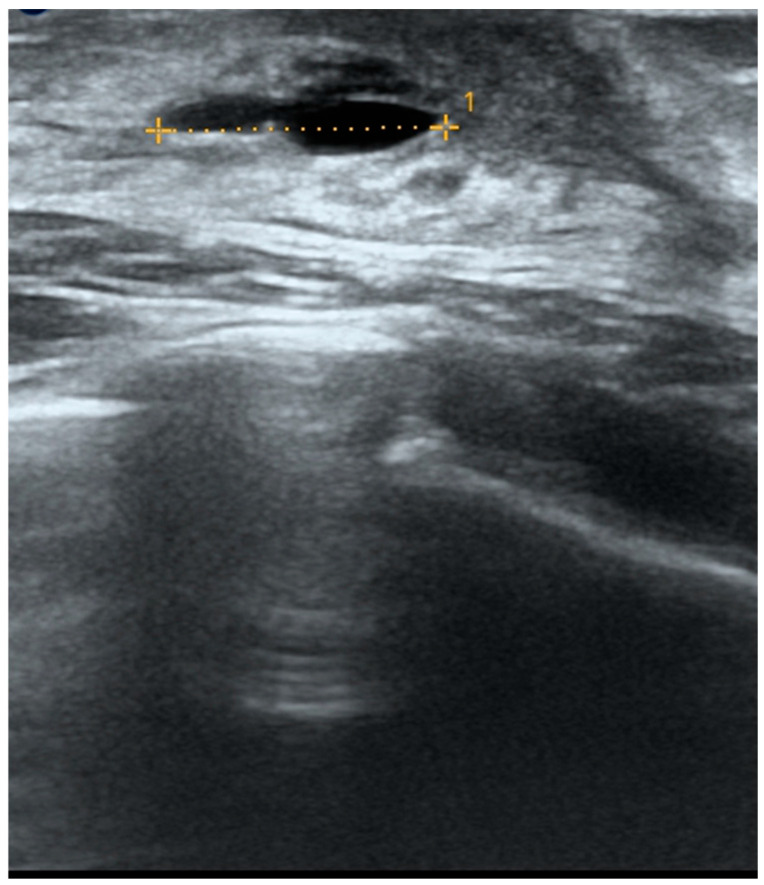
Baseline ultrasound (T0) showing an anechoic lesion with well-defined, regular margins (maximum diameter: 13 mm), consistent with a simple cyst.
